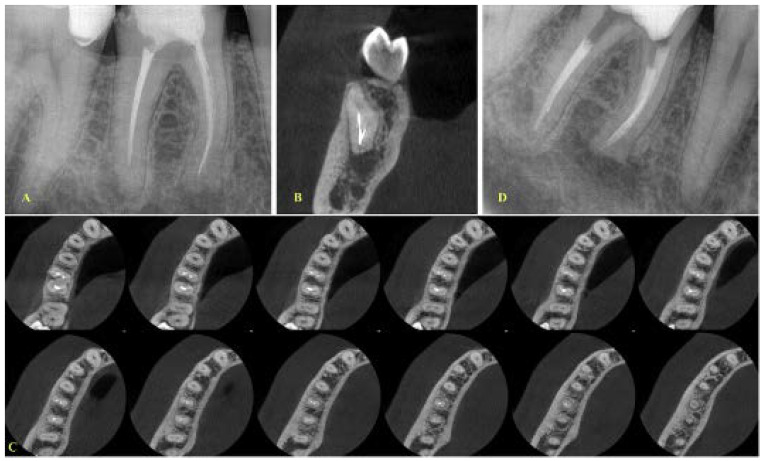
(A) Initial periapical radiograph of a mandibular first molar with periapical lesions and a fragment at the apical third of the mesial root (mesiobuccal canal), (B,C). Coronal and axial views demonstrating that the two canals are merging at the apical third (D) Final radiograph with the fragment in place. No attempt was made to remove the fragment. Apical patency was achieved through the mesiolingual canal.
